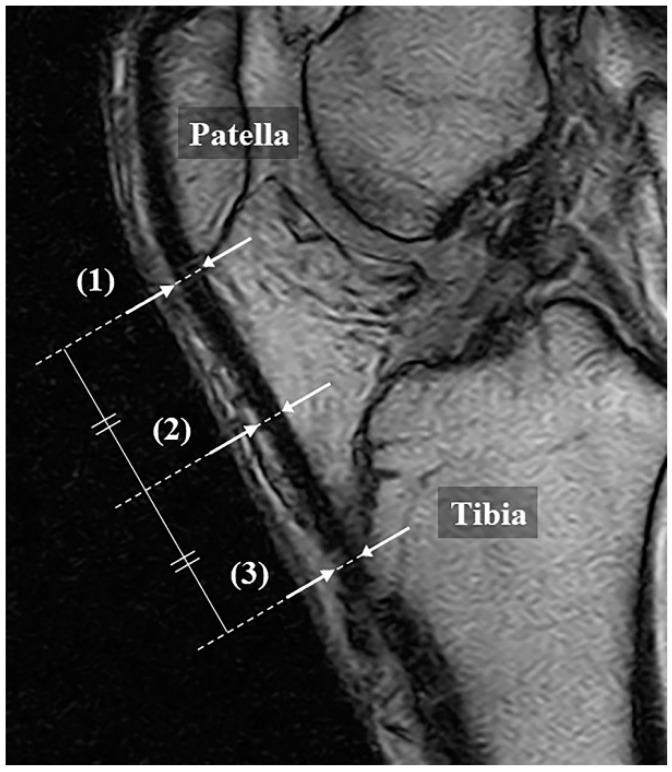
Measurement method of patellar tendon with MRI (gradient echo, sagittal). Thickness is defined as the distance from the superficial to the deep layers of the tendon at (1) the proximal part, (2) the midpoint, and (3) the distal part, respectively. This follows the method explained in Figure 1 for the ultrasonography.
